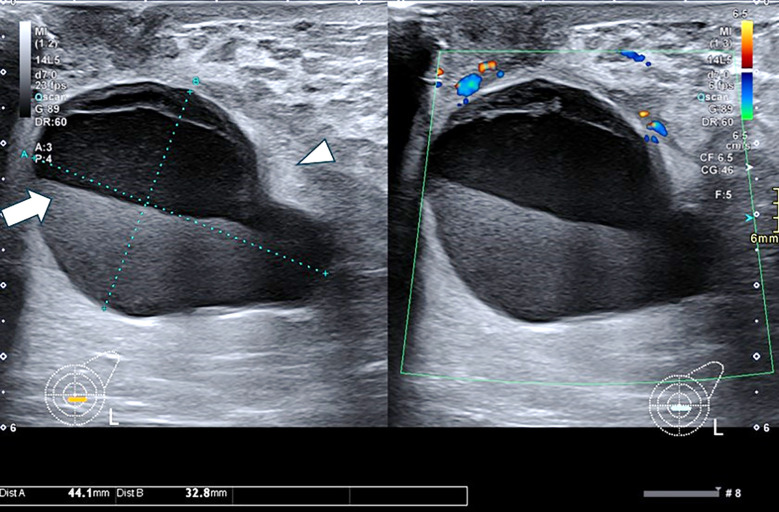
Ultrasound images of a young lactating female with a painful breast lump showing a cystic lesion with layering debris (arrow). The surrounding parenchyma shows increased echogenicity (arrowhead) and vascularity in the retroareolar region at 6 o’clock of left breast. Aspiration yielded milky fluid mixed with pus, consistent with an infected galactocele.
